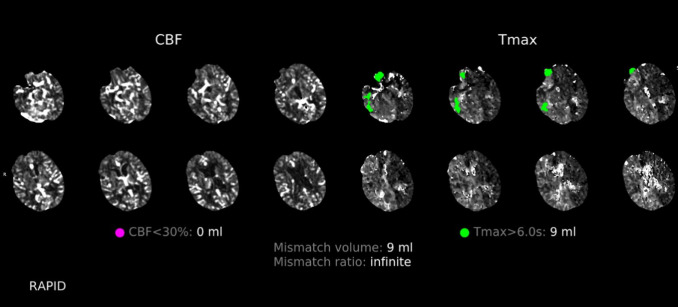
Computed tomography head with contrast demonstrating right frontal, parietal, and temporal regions with perfusion deficits (green). These deficits are defined by a time-to-maximum delay of over six seconds for contrast to move to the tissue. Without intervention, these regions are a reliable estimate of the final infarction area. There are no regions with cerebral blood flow deficits (purple), which would indicate completed ischemia.^4^ *CBF*, cerebral blood flow; *Tmax*, time to maximum.
